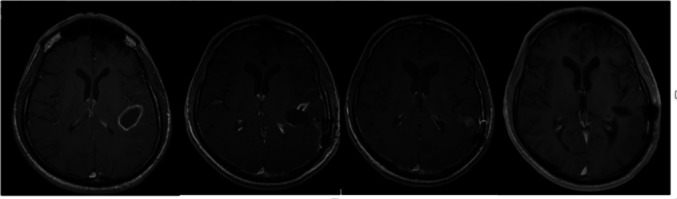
Sequential axial post-contrast T1- weighted MRI images obtained in a 72-year-old patient with right frontal brain abscess. A: Detection of a large abscess formation with intraventricular rupture, B: MRI performed on postoperative-day 1 after craniotomy, abscess evacuation and partial resection, C: MRI performed 20 days after initiation of HBOT showing further remission, D: MRI at 6-weeks follow up demonstrating no residual abscess
